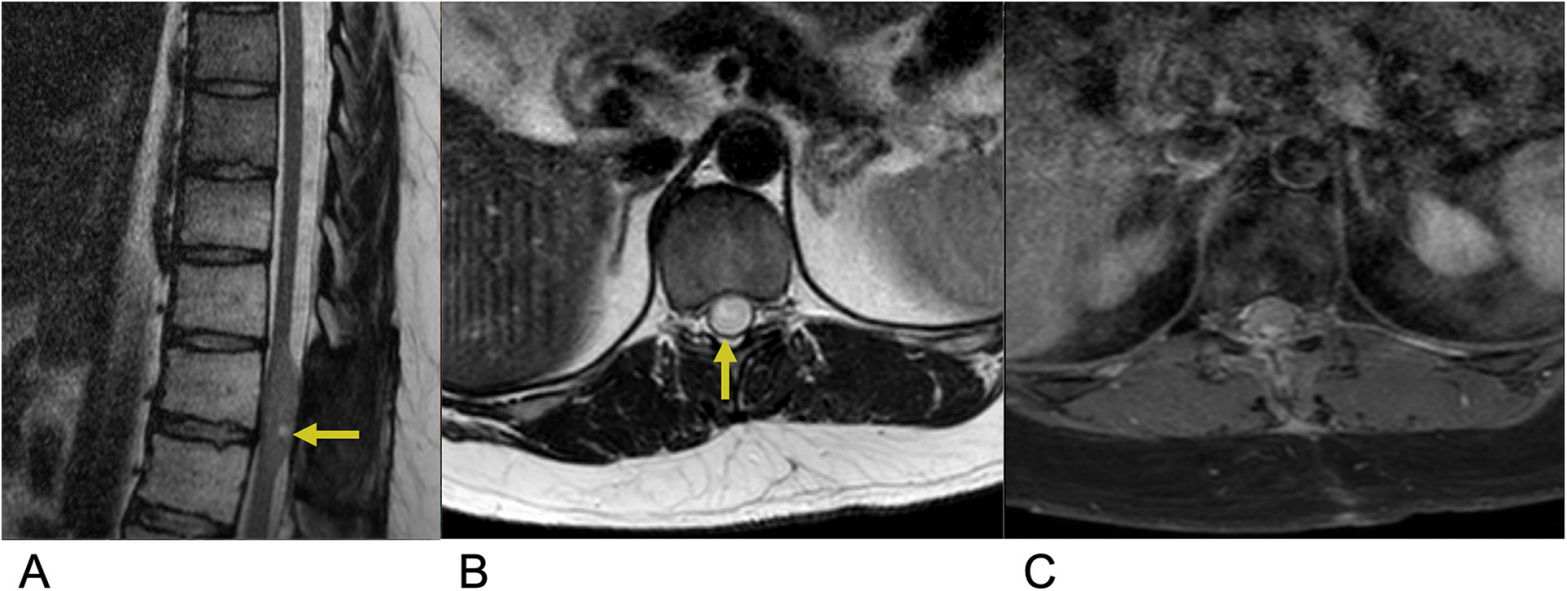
Astrocytoma. Sagittal T2 (A), axial T2 (B), axial T1 FS + C (C) MRI of the thoracic spine demonstrating an intramedullary expansile T2 hyperintense mass (yellow arrows) in the thoracic cord at the T10–T11 level without post-contrast enhancement representing biopsy-proven Grade 1 Pilocytic Astrocytoma.
